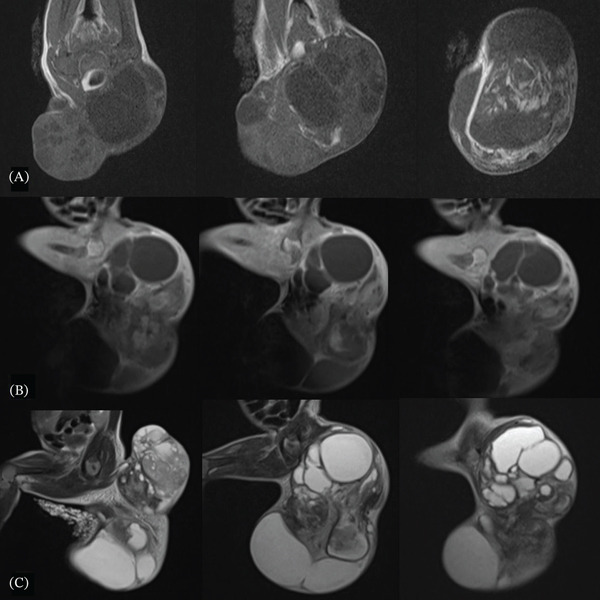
Multiplanar MRI of the left posterior humeral midshaft demonstrating a pedunculated osteochondroma. (A) T1‐weighted coronal image showing a lobulated osseous protuberance arising from the posterior aspect of the midshaft humerus, with continuity to the medullary cavity. (B) T2‐weighted coronal image highlighting the three‐lobed configuration of the lesion and the cartilage cap. (C) Fat‐suppressed axial T2‐weighted image demonstrating the cartilage cap thickness (~4 mm) and soft tissue interface.
